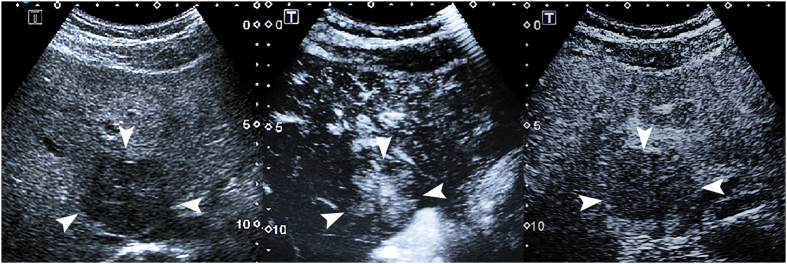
Focal nodular hyperplasia in a 29-year-old man. (a) A 5.4-cm lesion (arrows) in the liver shows hypoechogenicity on conventional ultrasound. (b) Contrast- enhanced ultrasound shows spoke-wheel arteries (arrow) in the lesion 12 s after contrast agent administration. (c) The lesion (arrows) shows hypoenhancement in comparison with adjacent liver tissue 69 s after contrast agent administration.
